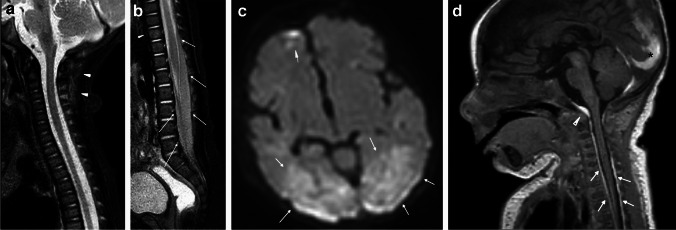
Two-month-old boy presenting with lethargy and possible seizure. Sagittal STIR MR image (a) of the upper spine demonstrates ligamentous edema in the nuchal ligament (*arrowheads*) and multiple interspinous ligaments. Sagittal STIR MR image (b) of the lower spine reveals the intermediate signal spinal SDH along the distal thecal sac (*arrows*). There is subtle marrow edema in the T10 vertebral body (*arrowhead*) consistent with contusion-type injury without loss of vertebral body height. Axial diffusion-weighted image (c) from a brain MRI demonstrates multifocal areas of diffusion restriction (*arrows*) compatible with areas of cytotoxic edema suggesting HII. A sagittal T1-weighted MR image (d) through the cervical spine obtained 1 week after presentation for ongoing seizures demonstrates redistribution of SDH with increased volume of T1-hyperintense subdural hemorrhage along the posterior falx (*asterisk*), in the posterior fossa, in the retroclival space (*arrowhead*), and within the upper spinal canal (*arrows*)
